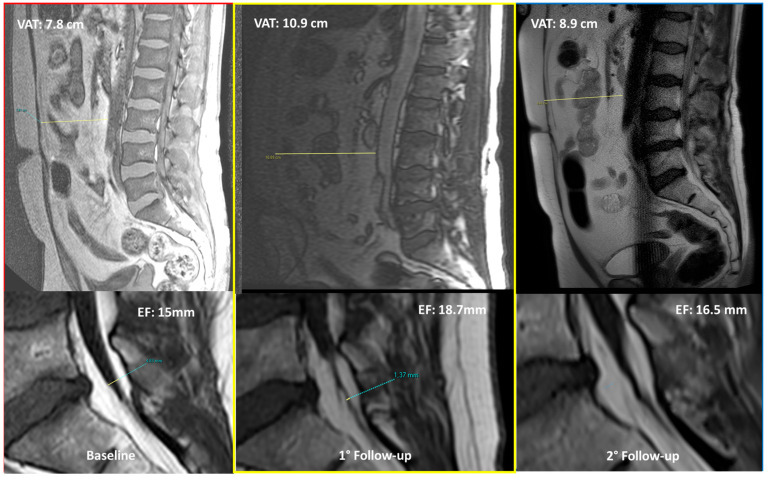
MRI controls over time (baseline, 1° follow-up, and 2° follow-up) in a middle-aged man affected by spinal epidural lipomatosis (with neurologic symptoms) and metabolic syndrome (with moderate overweight)—MR-localizer sequences (up), T1w sagittal sequences (down). VAT = visceral adipose tissue (cm). EF = epidural fat (mm). Patients’ weight at the moment of follow-up controls (1° MRI = 76 kg, 2° MRI = 87 kg, 3° MRI = 83 kg).
